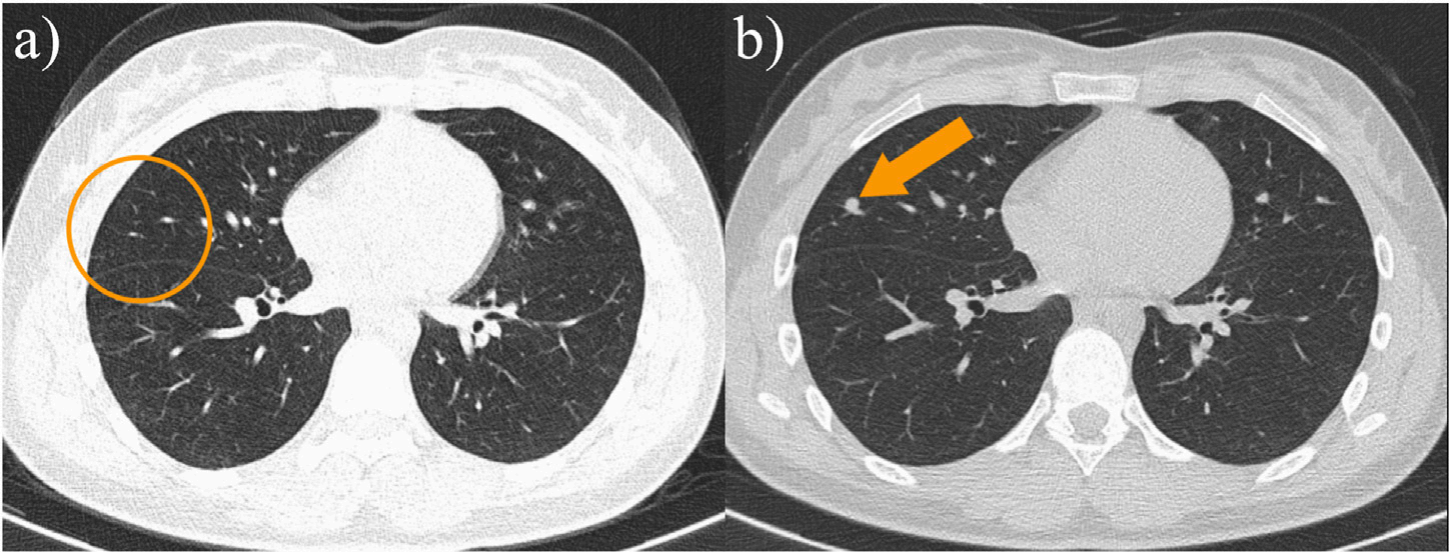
(A) The patient’s chest CT at the time of initial diagnosis did not reveal a definite metastatic lesion; (B) The patient’s follow-up chest CT 13 months after surgery showed the appearance of a new paravascular soft tissue nodule, and lung metastasis was considered.
